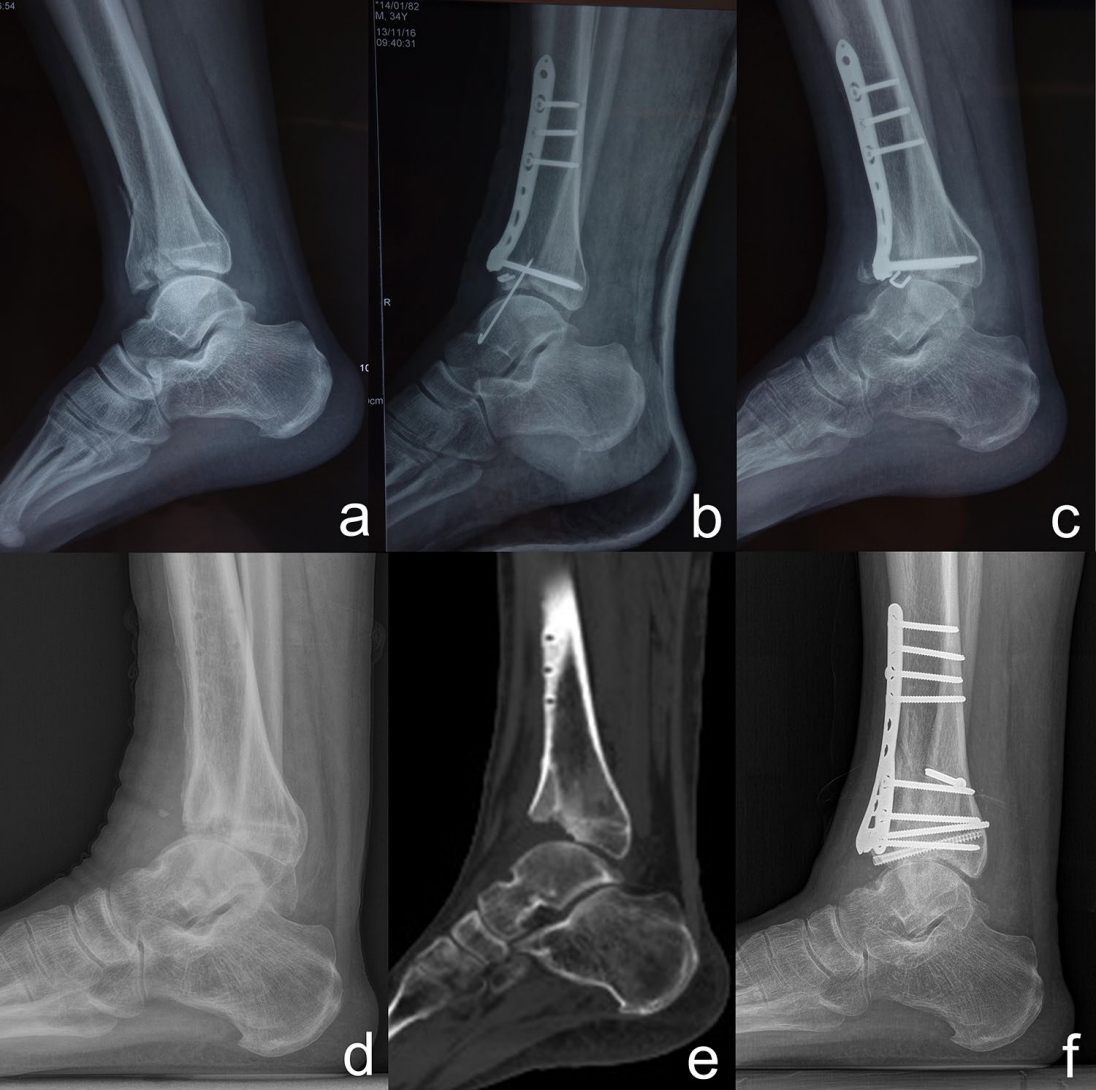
(a) A 36-year-old man sustained a pilon type B ankle fracture. (b) A congruent ankle joint and anatomical reduction of the fragments were observed on X-rays immediately after ORIF. (c) This patient gradually developed anterior subluxation of the ankle joint and impaction of the anterior distal tibial plafond. (d–e) Twelve months after the initial surgery, this patient visited our clinic for consultation because of worsening pain around the ankle. (f) At 16 months after the revision operation, alignment of the ankle was normal on the sagittal plane, and the patient was satisfied with the results of the operation.
